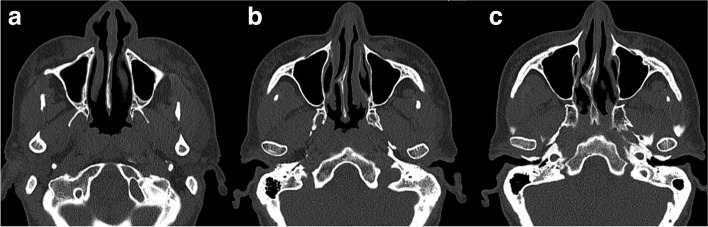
CT of the external nose and nasal cavity of the patient B, depicting the following: a thickened and irregular mucosal lining of septum; b collapsed internal valves bilaterally, septal spur with septal deviation, and medialized left lower turbinate; c large septal spur located posteriorly, asymmetric short nasal bones, right pointed inwards, left pointed more perpendicularly, and asymmetry of surrounding facial features
